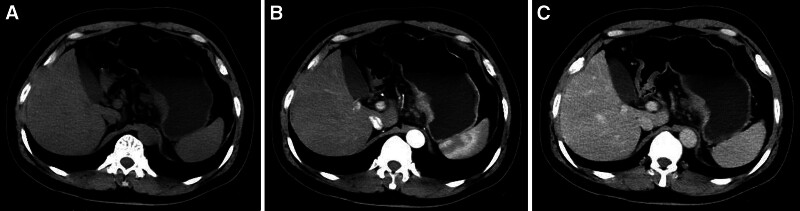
A typical case (male, 52 years old, nerve invasion (+)) with local gastric wall thickening with abnormal enhancement on CT (T3 stage, N0 stage, adenocarcinoma, nonpoor differentiated, mixed type). (A) Plain CT image: local gastric wall thickening in the gastric cardia. (B and C) Enhanced CT image: the thickened gastric wall in the gastric cardia is significantly enhanced.
